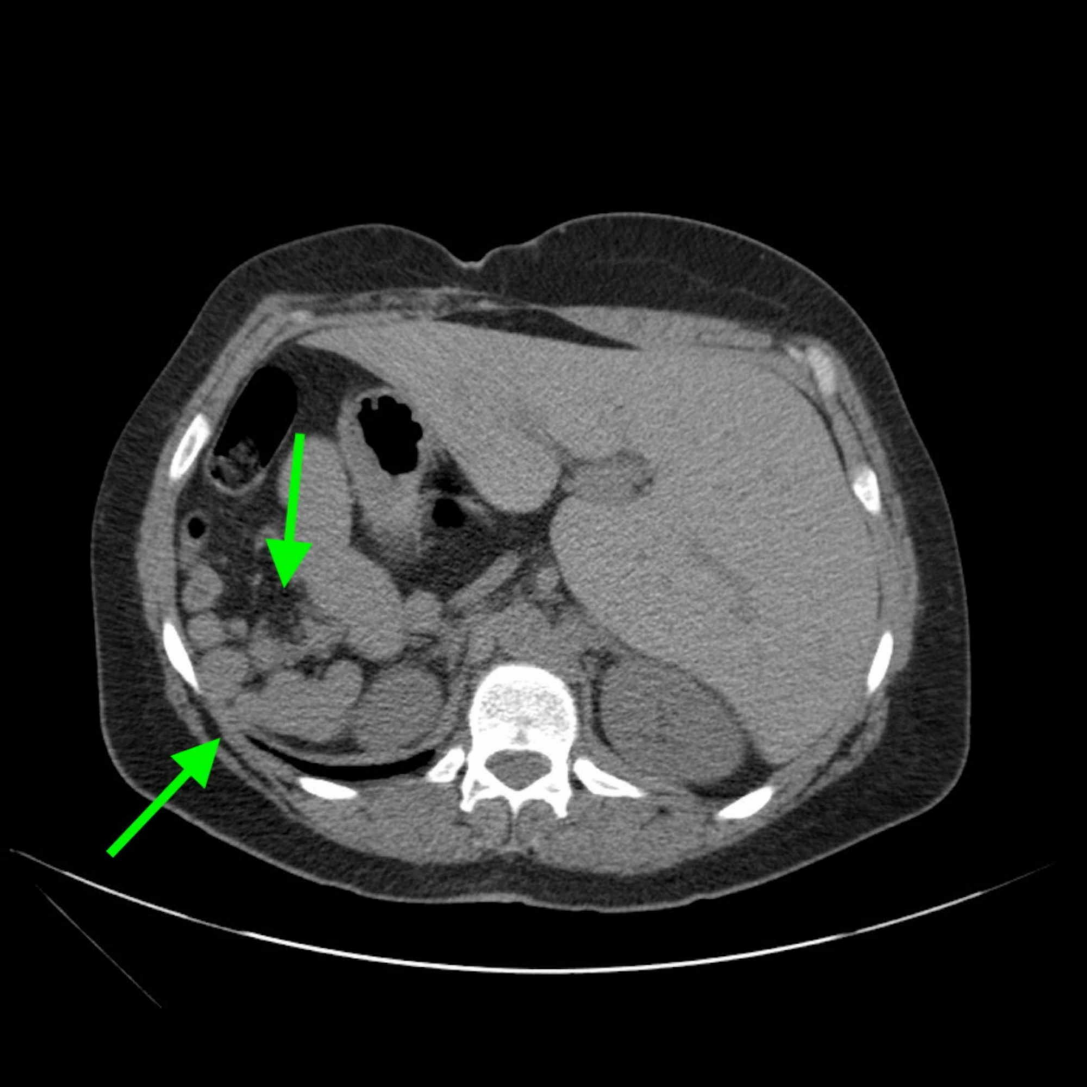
Splenules noted CT abdomen and pelvis.
